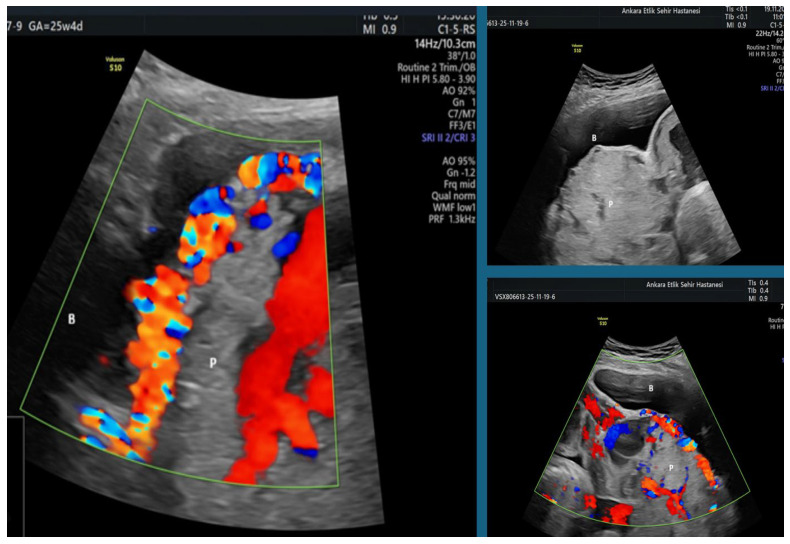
Ultrasound findings of a PAS 2–3 case demonstrating uterovesical hypervascularization, placental bulging toward the bladder, and multiple irregular placental lacunae on a combination of gray-scale and color Doppler imaging (B: Bladder; P: Placenta).
